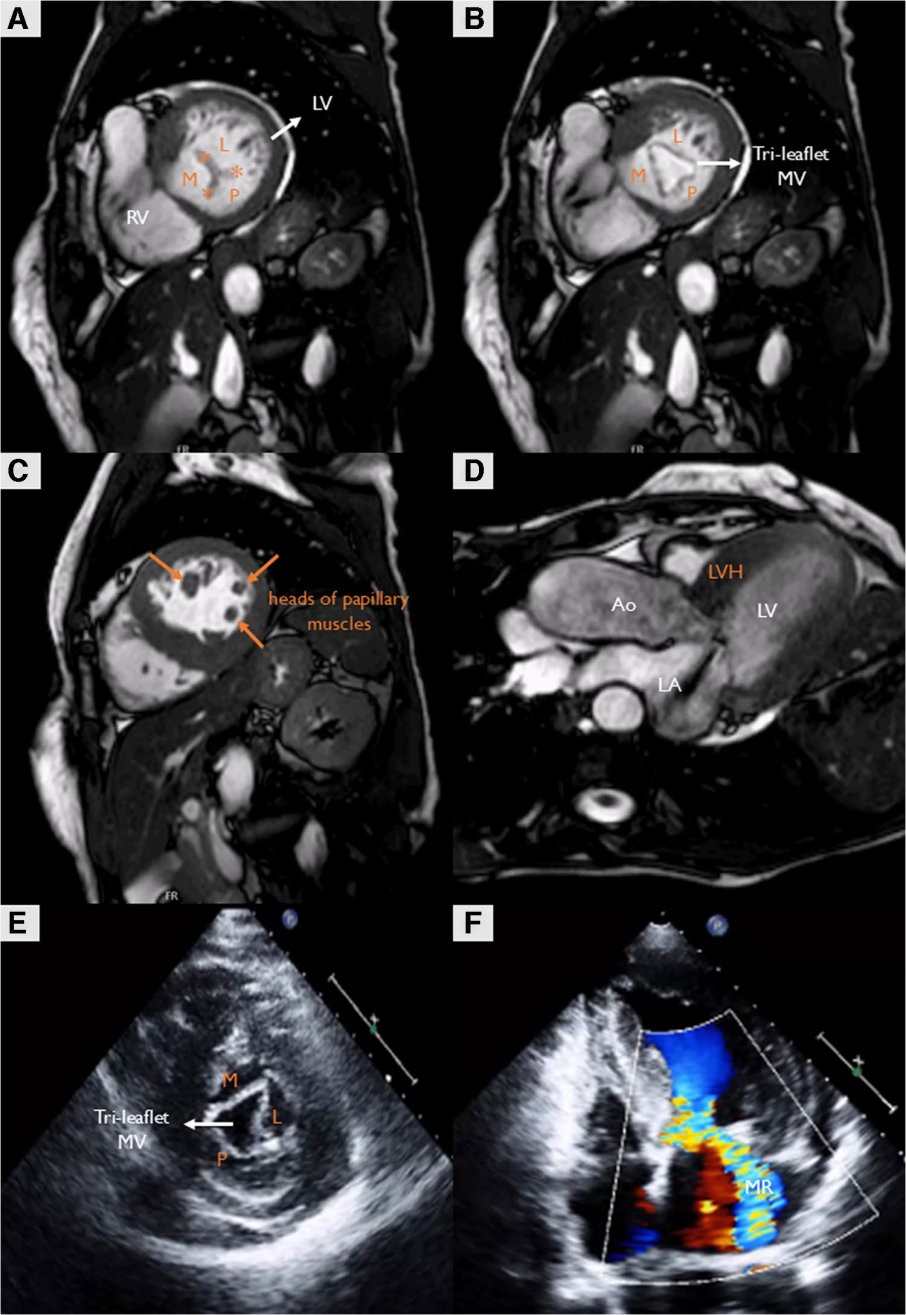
Multimodality imaging of a tri-leaflet MV in HOCM. A–C Still frames of cine CMR (steady-state free precession sequence; short-axis views), demonstrating a tri-leaflet MV in closed (A) and open (B) positions, with the respective three anteriorly displaced papillary muscles (C). D Still frame of cine CMR (steady-state free precession sequence; three-chamber view) showing systolic anterior motion of the MV, with an eccentric MR jet directed to the posterior wall of the LA. E TTE (parasternal short-axis view) depicting a tri-leaflet MV in the open position. F Baseline TTE (apical four-chamber view) showing a moderate to severe, eccentric MR jet directed towards the lateral wall of the LA. Ao, ascending aorta; CMR, cardiac magnetic resonance; HOCM, hypertrophic obstructive cardiomyopathy; L, lateral leaflet of the MV; LA, left atrium; LV, left ventricle; LVH, left ventricular hypertrophy; M, medial leaflet of the MV; MR, mitral regurgitation; MV, mitral valve; P, posterior leaflet of the MV; RV, right ventricle; TTE, transthoracic echocardiography. Asterisks (*) indicate the three equidistant commissures. Orange arrows indicate the heads of the three anteriorly displaced papillary muscles
